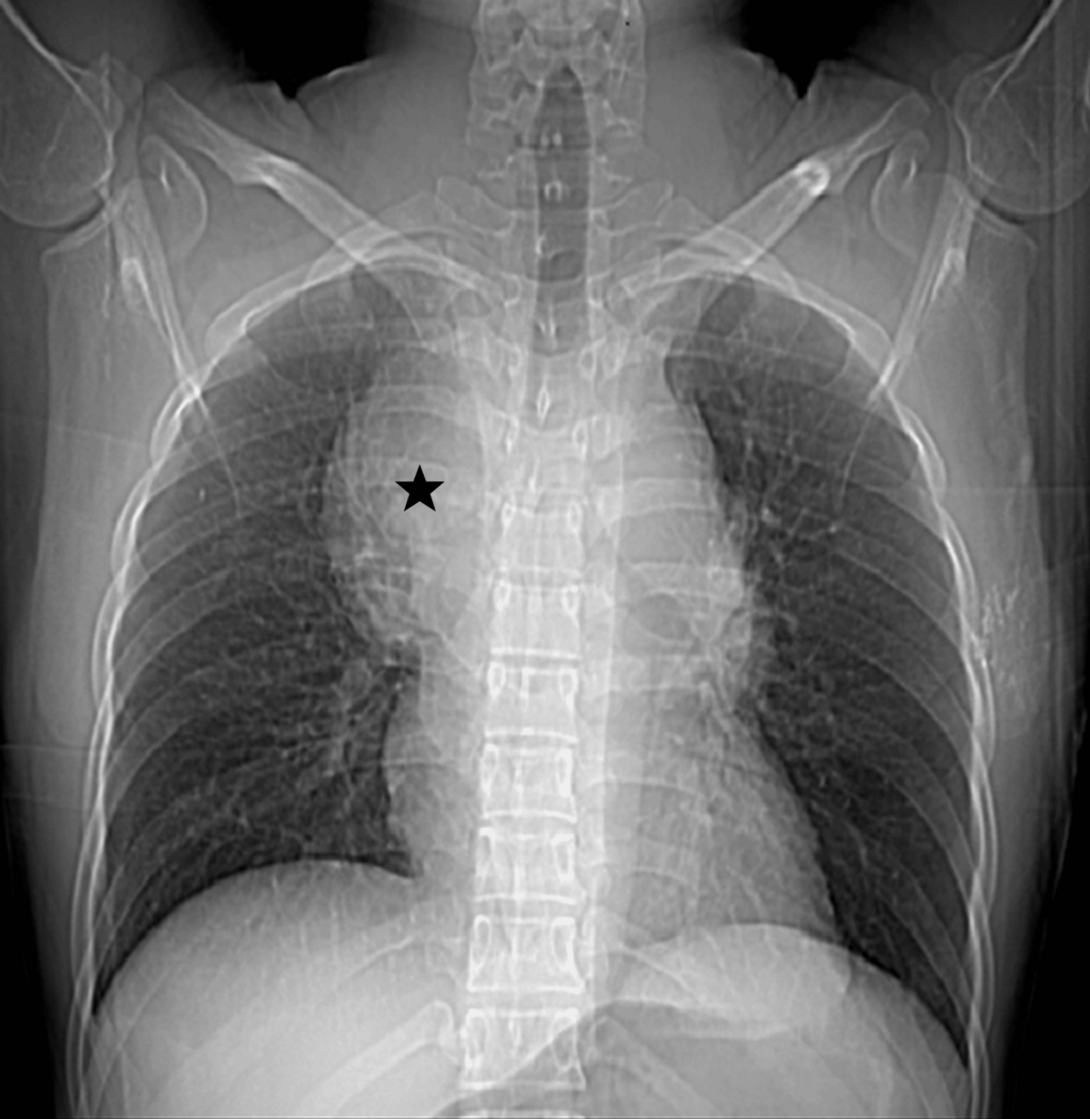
Chest X-ray showing mediastinal widening due to a mass (black star).
